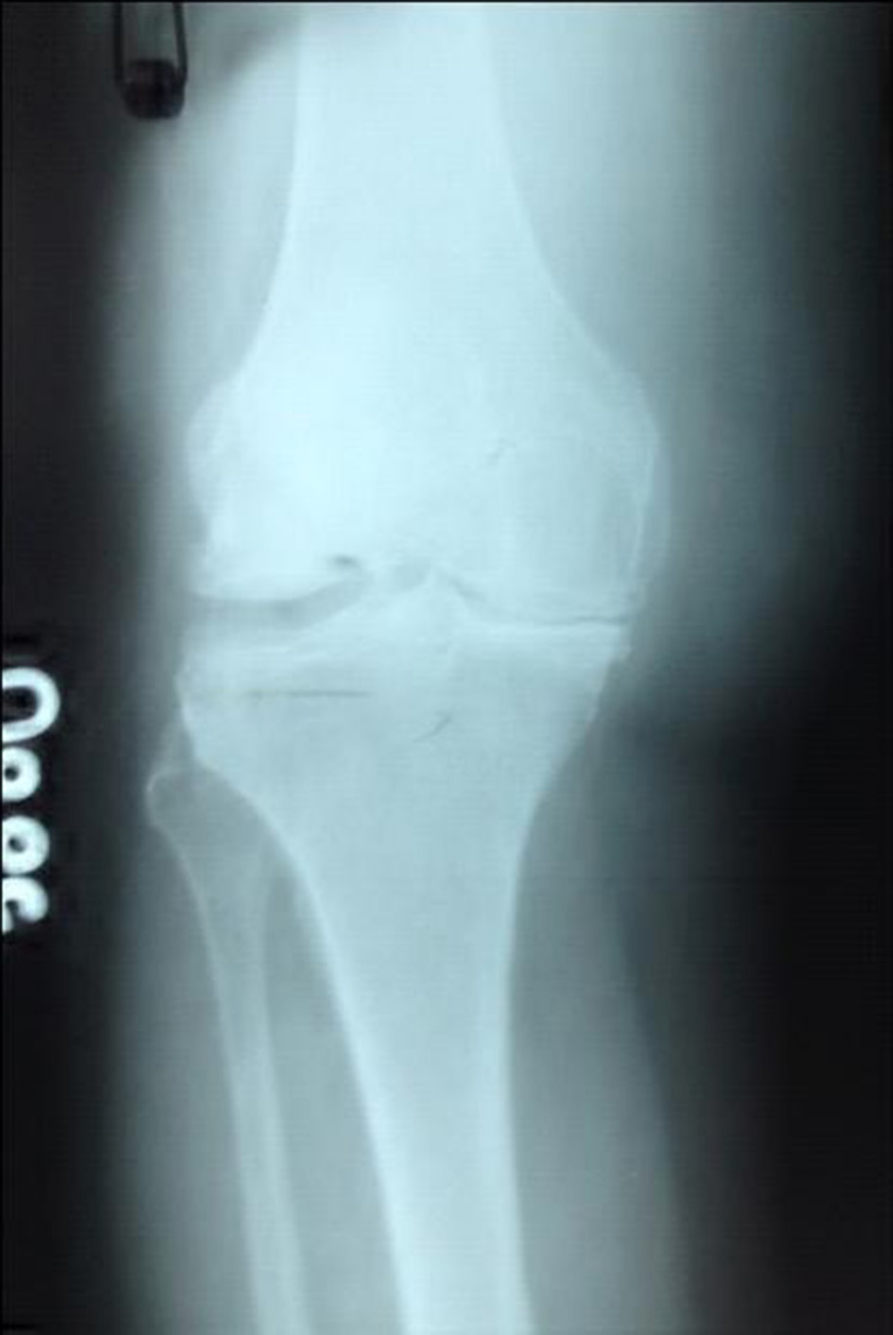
Knee Radiography Before Prolotherapy
